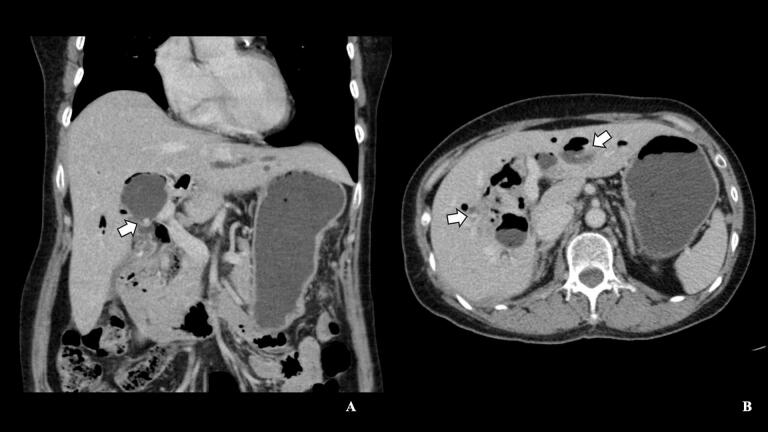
Postoperative CT scan at 6-month follow-up. (A) Coronal and (B) axial contrast-enhanced CT images at 6-month follow-up. Small retained stones (white arrows) are visible, but there is no significant biliary obstruction. The patent choledochoduodenostomy can be appreciated, indicating successful long-term biliary drainage.
